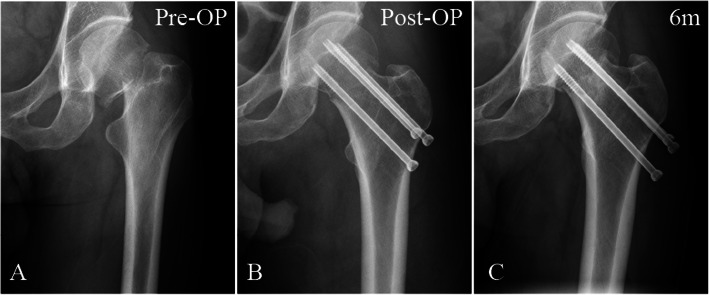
AP radiographs of a parallel screw fixation in a 58-year-old man. a Preoperative radiograph showing a vertical femoral neck fracture. b Postoperative radiograph showing treatment with three cannulated screws. c Radiograph taken 6 months postoperatively in the same patient, showing severe screw withdrawal, femoral neck shortening, and delayed union, as seen in the fracture line. *Pre*-*OP* preoperative, *Post*-*OP* postoperative, *6 m* 6 months after surgery
